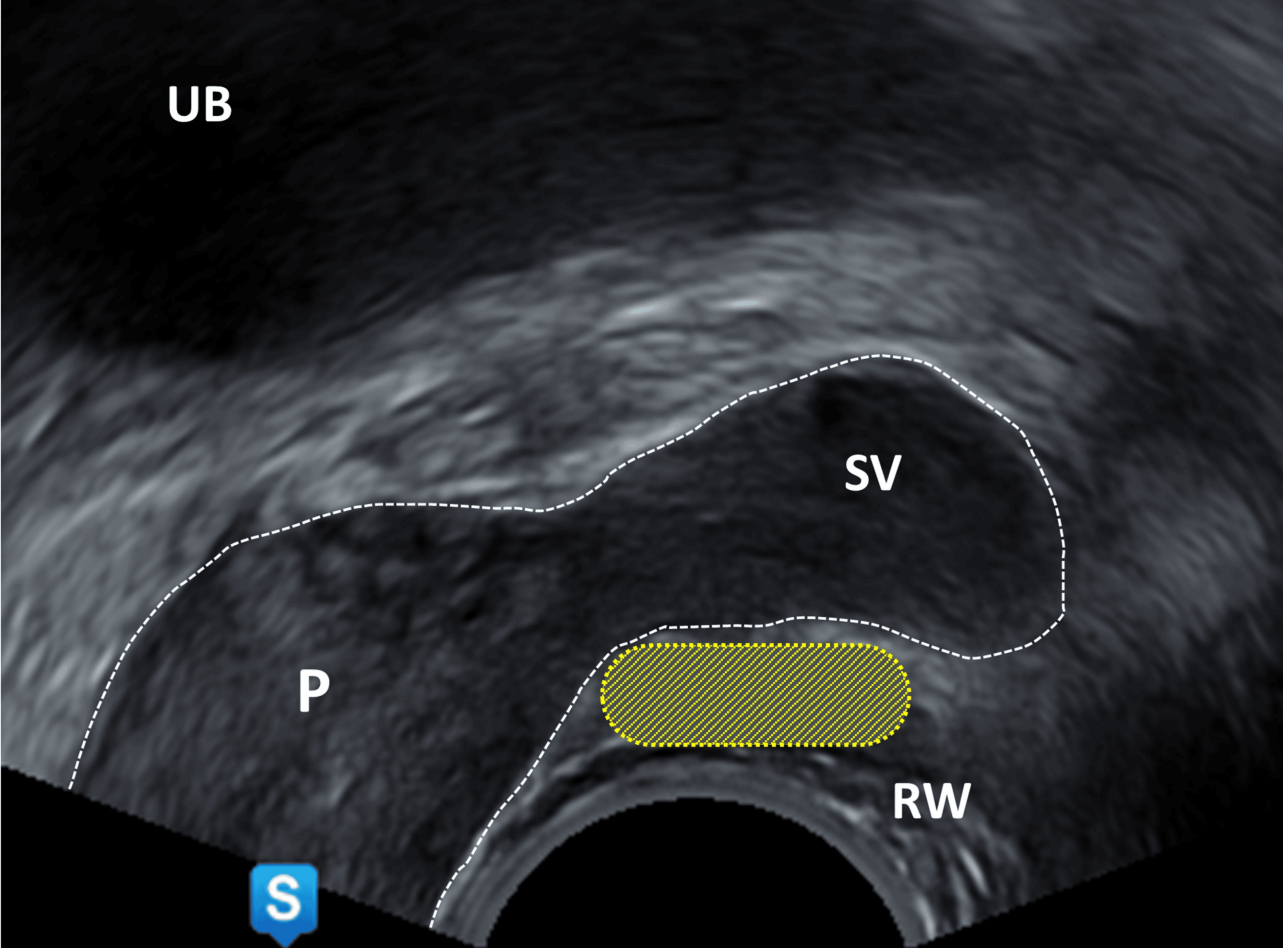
Sagittal section of the prostate, ultrasound probe slightly rotated Yellow area: area of interest for local anesthesia  between SV, P, and RW UB: urinary bladder, P: prostate, SV: seminal vesicle, RW: rectal wall Image Credit: Toni Franz, corresponding author
